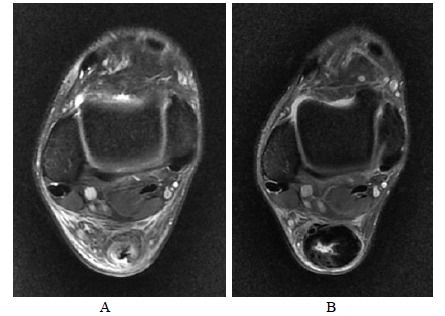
2 months and 6 months after open surgery for Achilles repair in Patient A. Fat suppressed T2-weighted axial images show replacement of internal hypointense scar tissue at the operative site in a centripetal fusion.
